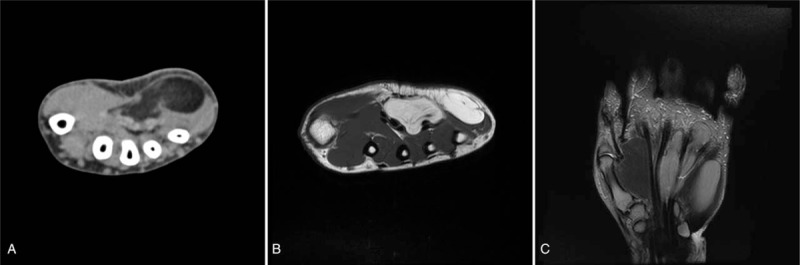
Preoperative computed tomography (A) and magnetic resonance imaging (B, C) scans of the mass. Preoperative image studies revealed an encapsulated and multilobulated mass compatible with a lipoma, which measured 8 cm × 5 cm × 2 cm.
